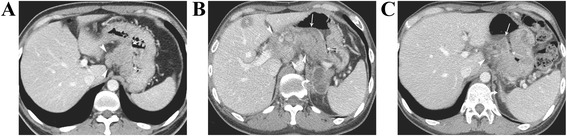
a. A contrast-enhanced abdominal computed tomography (CT) image shows enhancing gastric cancer (arrows) of the gastric body and conglomerate metastatic lymphadenopathies (arrowheads) along the left gastric chain. b. A follow-up contrast-enhanced abdominal CT image demonstrates aggravated gastric cancer of the gastric body (arrows), multiple metastatic lymphadenopathies (arrowheads) in the gastrohepatic space, a hepatic metastasis (asterisk), and left adrenal metastases (curved arrow). c. A follow-up contrast-enhanced abdominal CT image after anti-PD-1 therapy shows improving gastric cancer (arrows) of a swollen gastric body and markedly decreased metastatic lymphadenopathies (arrowhead). Note the resolution of the previous metastases in the left adrenal gland (curved arrow)
